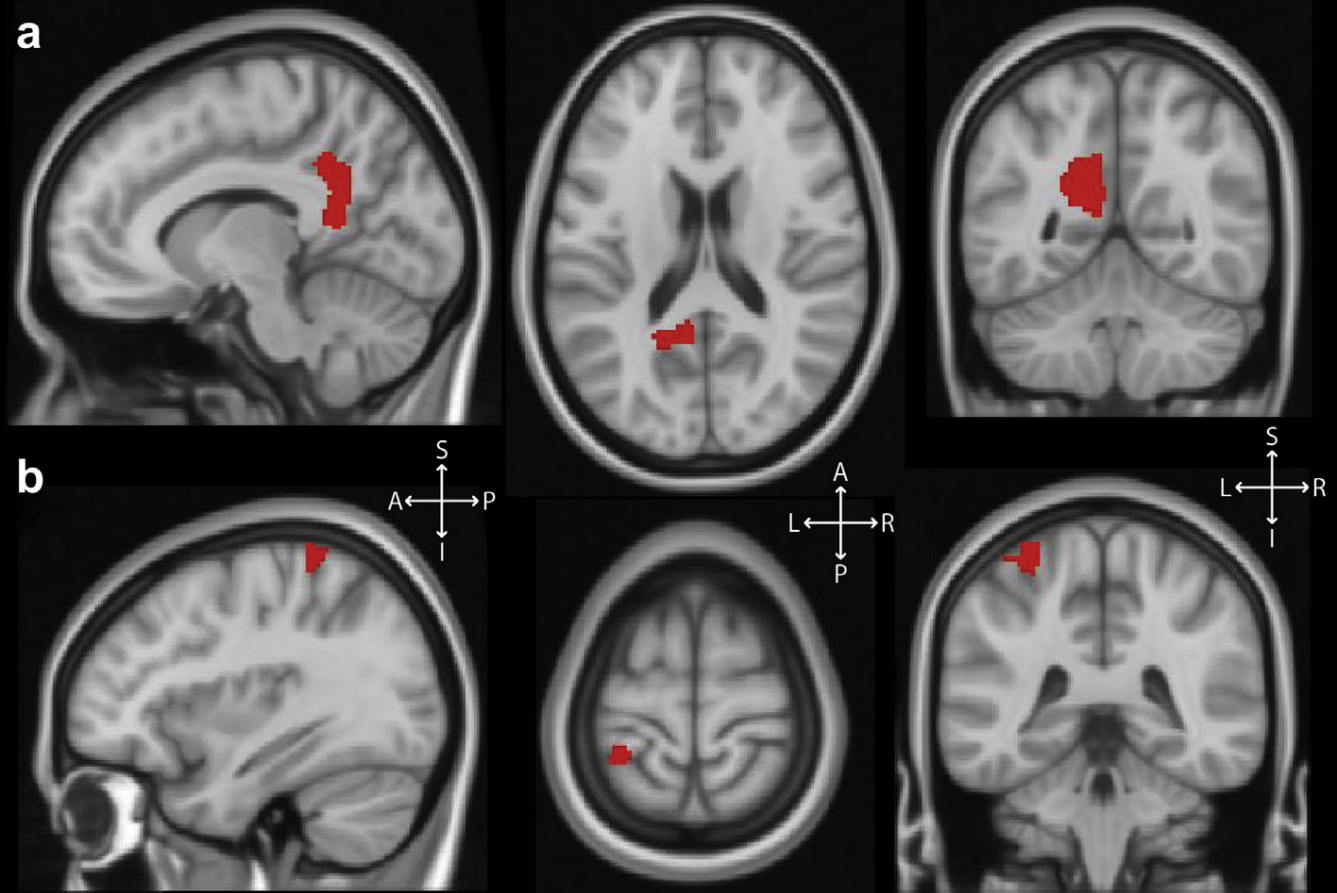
Beta band (14–30 Hz) oscillatory power increases (red areas) associated with increased (high *vs* low) hallucination intensity, displayed on saggital (left), axial (middle) and coronal (right) sections of a standard template MRI scan. a: Left posteromedial cortex, comprising a combination of posterior cingulate cortex, precuneus and retrosplenial cortex. b: Left primary motor cortex corresponding to the right arm/hand area. Abbreviations: S = superior, I = inferior, A = anterior, P = posterior, L = left, R = right.
